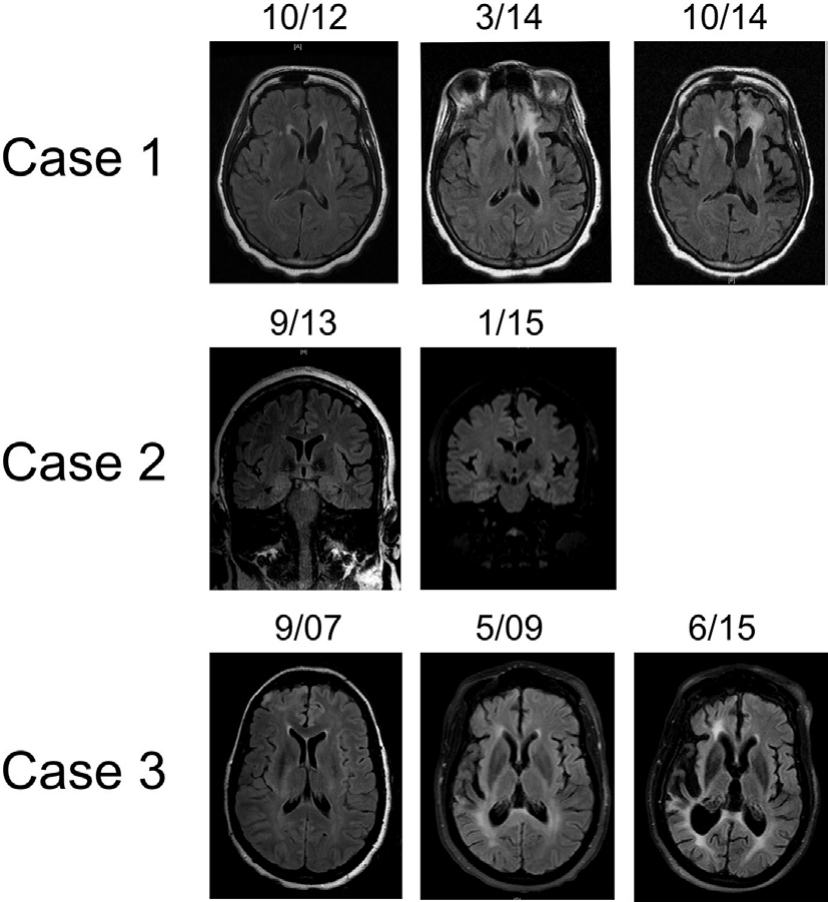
Representative T2 FLAIR MR images highlighting hemispheric atrophy over time. Patient 1 (top panel) showed left frontal predominant atrophy over a 2-year period; axial sections. Patient 2 (middle panel) displayed focal left frontal atrophy over an 18-month period; coronal sections. Patient 3 (bottom panel) had profound right cortical atrophy over a 7-year period; axial sections. MR images were obtained on machines using a 3-T magnet.
